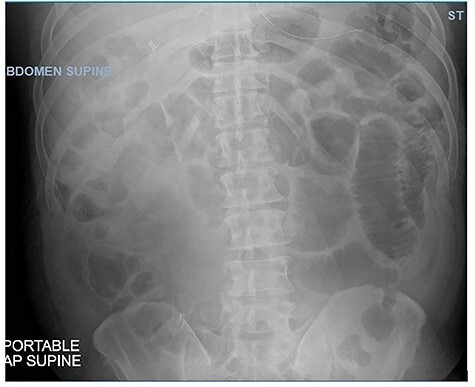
Abdominal X-ray showing dilated loops of bowel on Day 9 of admission.
